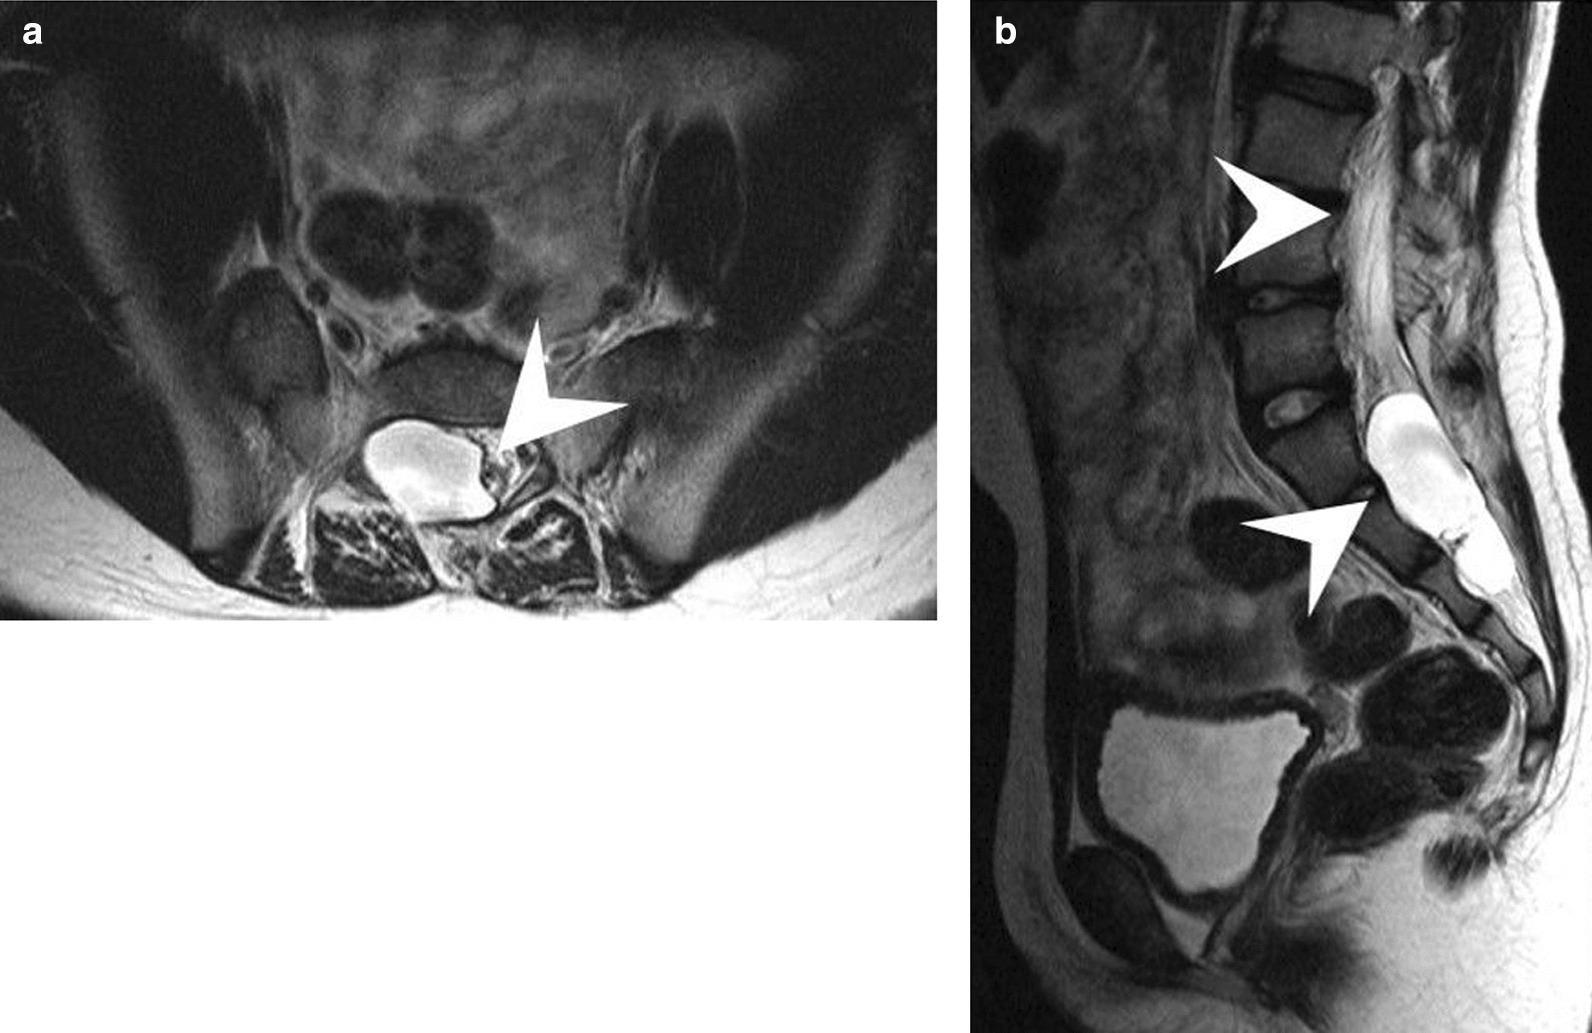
A T2 spine MRI demonstrated an S1–3 perineural cyst about 1.8 cm in length and 0.5 cm in diameter with no nerve root within the cyst. Arrowheads pointing the perineural cyst. B T2 lumbar spine MRI revealed low lying conus at the L3–L4 interspace with stretching of the filum terminals to the posterior dura wall at the S1–S2 level. upper arrowhead: Low lying conus, lower arrowhead: Posterior dura wall
